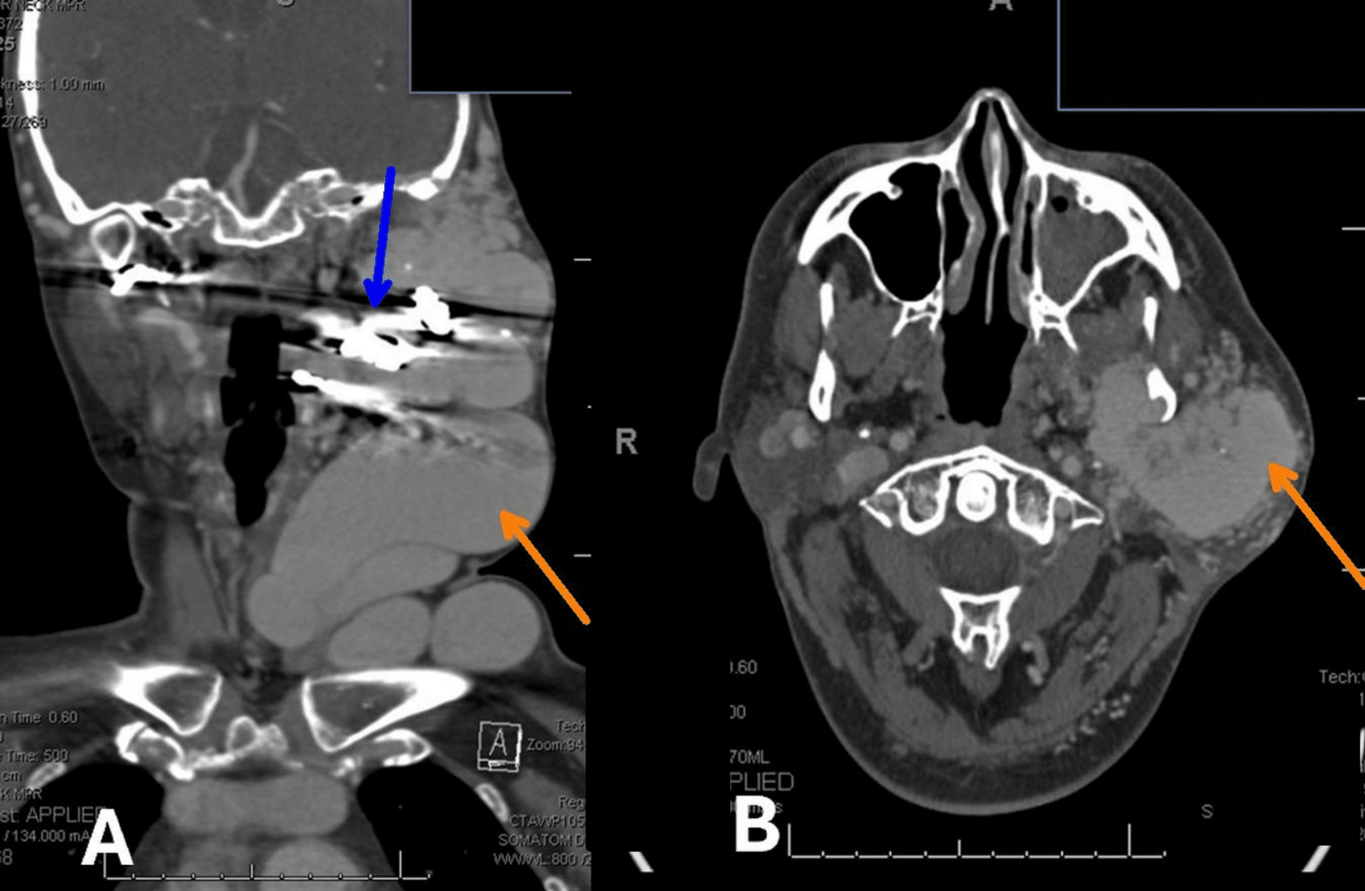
Contrast-enhanced CT angiography of the head and neck. Image A is a lateral view and shows some contrast with stasis from blockage going toward the large mass, which is getting minimal, if any, flow post-embolization. The orange arrow indicates the region of prominent abnormal vascular channels corresponding to the AVM nidus. The lesion is centered in the left parotid gland, with extension into the left masticator space and inferiorly into the upper neck. The blue arrow indicates the main arterial feeder from the left external carotid artery. Image B is an axial view with orange arrow at large mass with a few specks of white residual blood left after embolization. Yellow area shows residual large mass involving cheek, tongue, or throat, and neck. There appear to be other lobular masses lower with no vascularity that may have arteries elsewhere.
